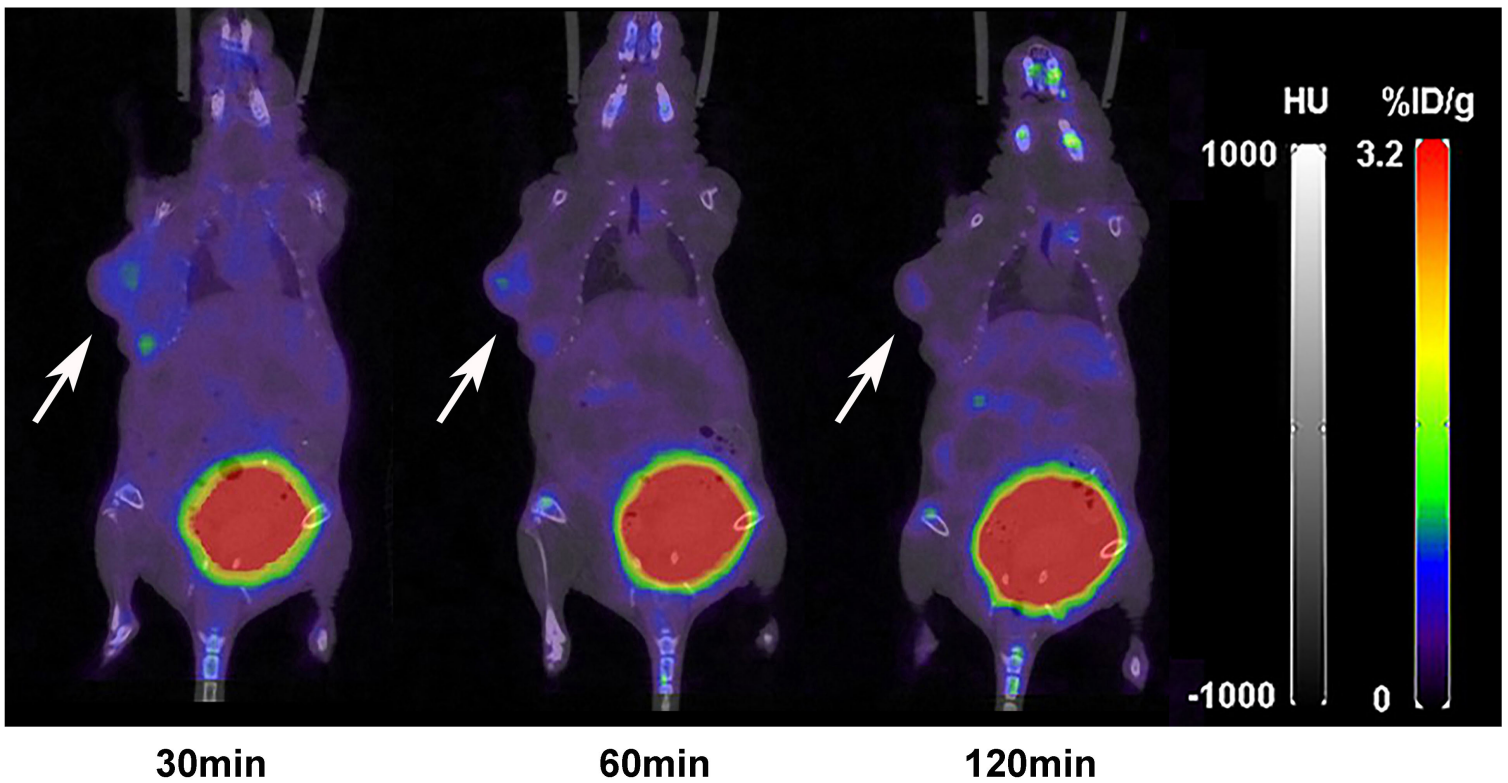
Micro-PET/CT imaging of RT-112 (FGFR1-high expression)-blocked xenografts injected with 11.1 MBq [^18^F]F-FGFR1 at different time points. The accumulation of [^18^F]F-FGFR1 in tumors were markedly decreased. Arrows indicate tumors.
